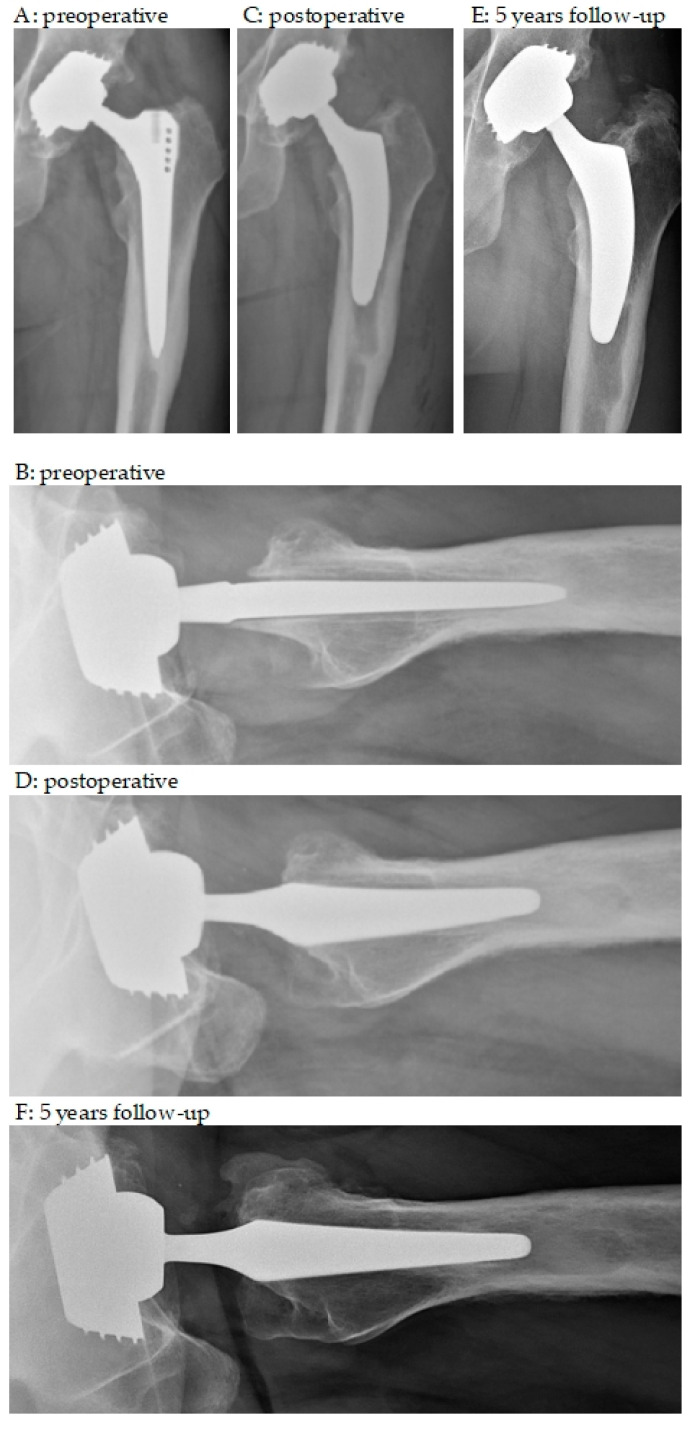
Anteroposterior and axial X-rays. (A,B) preoperative; (C,D) postoperative; (E,F) 5 years follow-up. A 52-year-old male with increasing pain and immobilization 6 years after THA; progressive stress shielding distally around the stem and loosening zone according to all Gruen zones; caused by aseptic loosening stem revision from a tapered rectangular stem to an short stem; in axial view, the relatively broad shoulder region compared to the explanted stem allows sufficient bone stock and provides superior rotational stability; from the first to last follow-up even new re-ossifications of the persisting loosening zones around the mid-stem.
